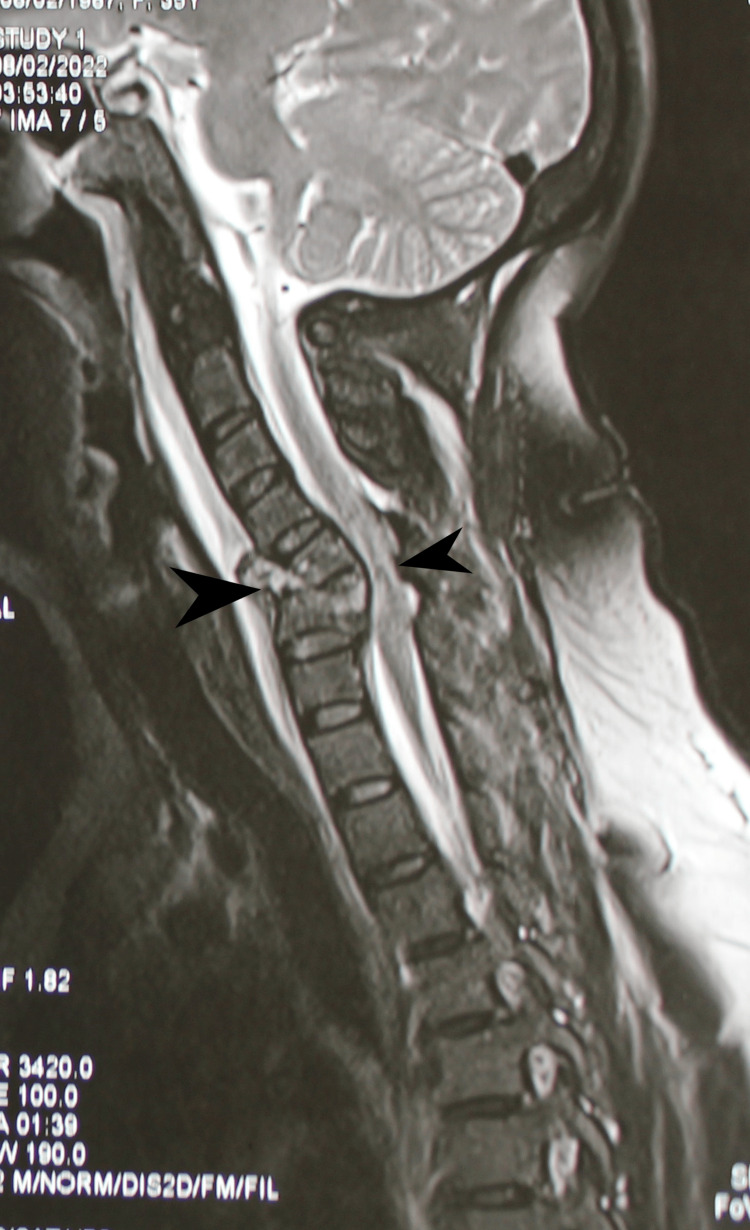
MRI cervical spine showing compression fracture of C5 &C6 vertebra with cord compression
